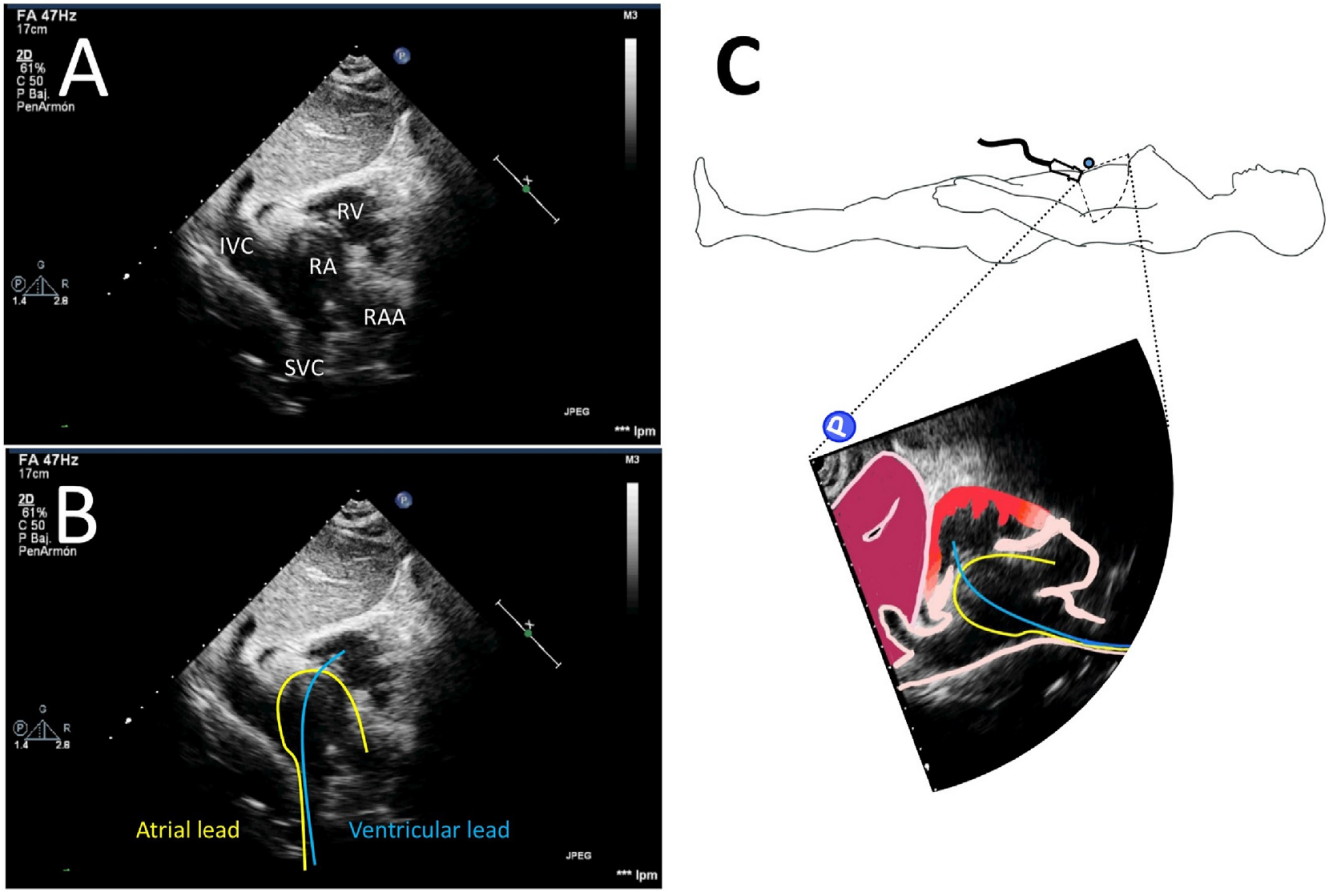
The figure A shows a subxiphoid window of the 2D transthoracic echocardiography taken intraprocedural. Figure B is for enhance the recognition of the leads in this view. Figure C shows a graphical representation of the position of the echocardiography probe and beam. Notice how the curve and tip of the atrial lead point toward the front of the patient. RV right ventricle, IVC inferior vena cava, RA right atrium, RAA right atrial appendage, SVC superior vena cava.
